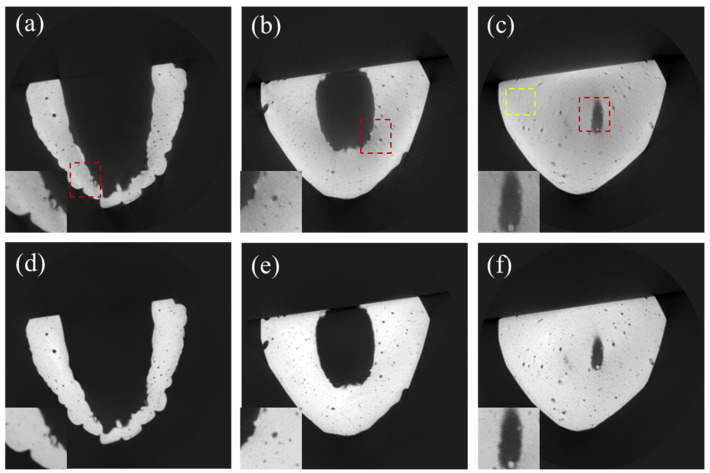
CT images of Model 3. (a–c) Uncorrected CT images. (d–f) DSC-SAC-corrected CT images. The zoomed images in the bottom left corner are related to the red ROIs while the yellow Roi is used to evaluate the signal to noise ratio (SNR).
